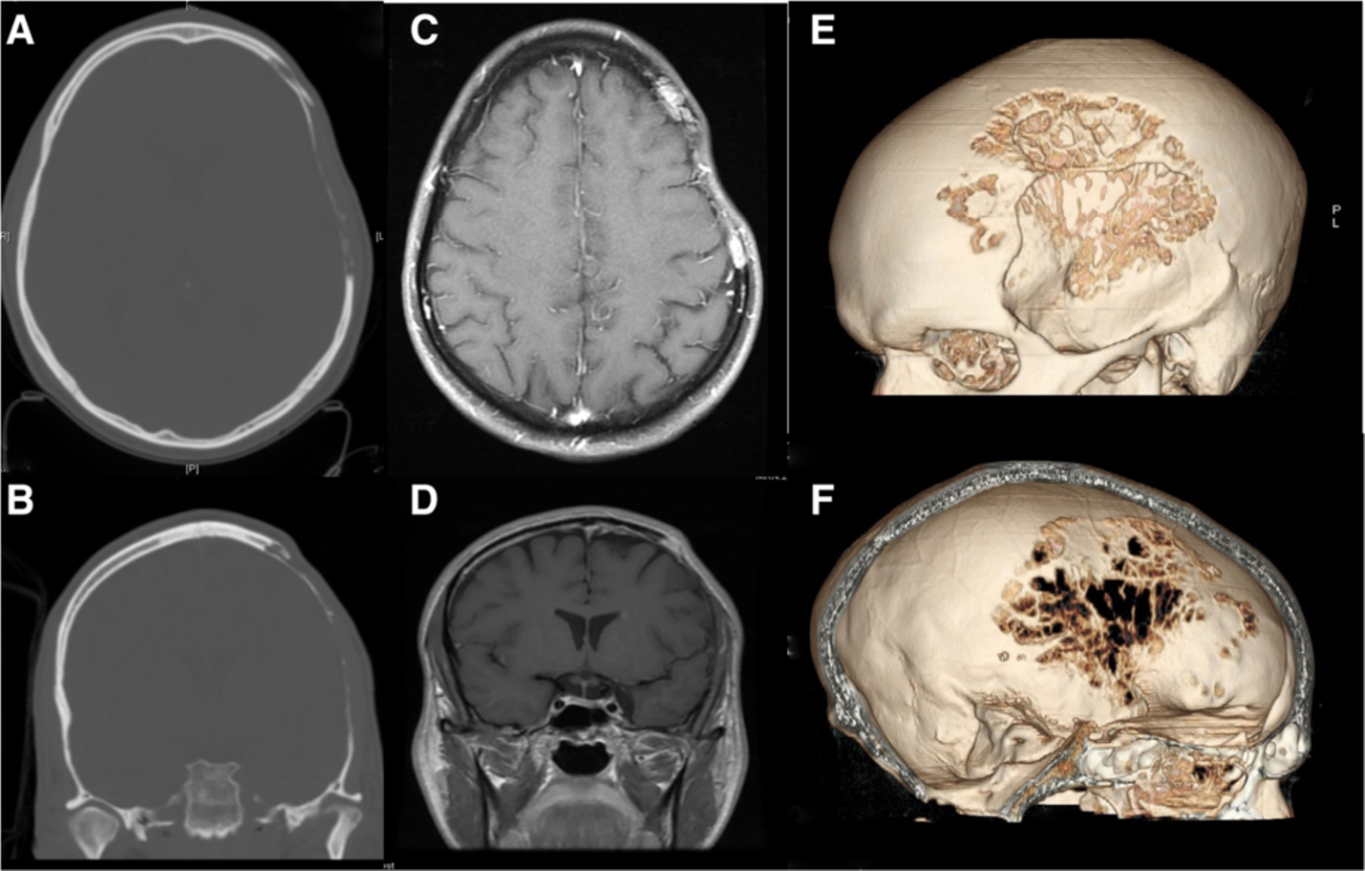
Preoperative images. A. and B. Preoperative CT of the skull bone window axial and coronal respectively showing frontparietotemporal bone thinning, erosion and defect. C. and D. Preoperative MRI brain T1-WI with contrast showing the marginal enhancement. E. and F. CT skull with 3D reconstruction showing the variable degrees of osteolysis from outside and inside respectively.
